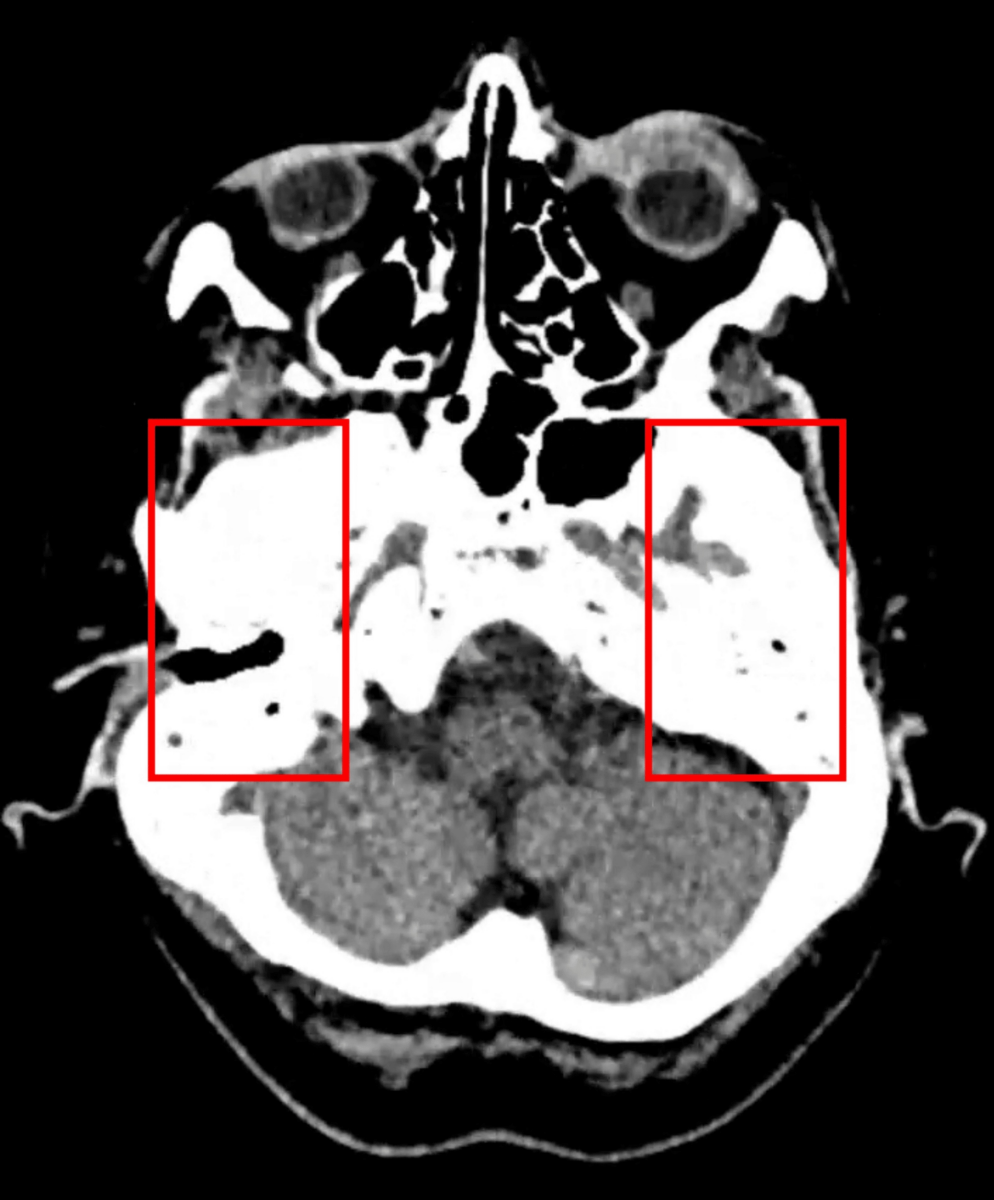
Axial CT showing soft tissue density in the external auditory canal Mild soft tissue debris is noted within the right external auditory canal, likely representing impacted wax or local inflammatory tissue.
